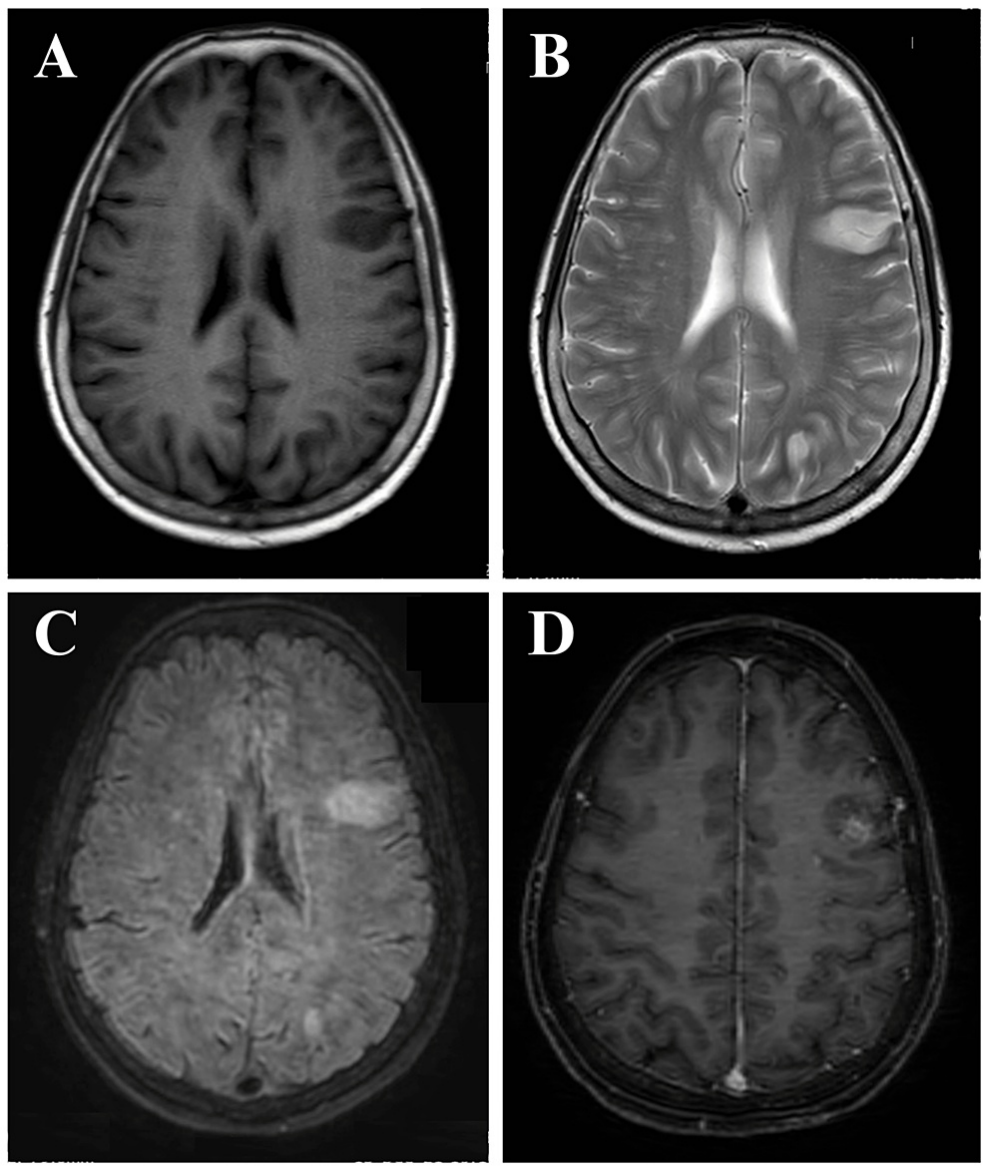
Axial T1W (3A), T2W (3B), FLAIR (3C), and post-gadolinium T1W (3D) MR images of the brain at different levels show multiple discrete T1 hypointense and T2 and FLAIR hyperintense lesions (arrows) of variable sizes in the periventricular and subcortical white matter of both cerebral hemispheres, with the largest lesion in the left frontal lobe involving the overlying cortex. A post-contrast scan shows nodular enhancement of the lesions (3D). T1W: T1-weighted; T2W: T2-weighted; FLAIR: fluid-attenuated inversion recovery; MR: magnetic resonance
